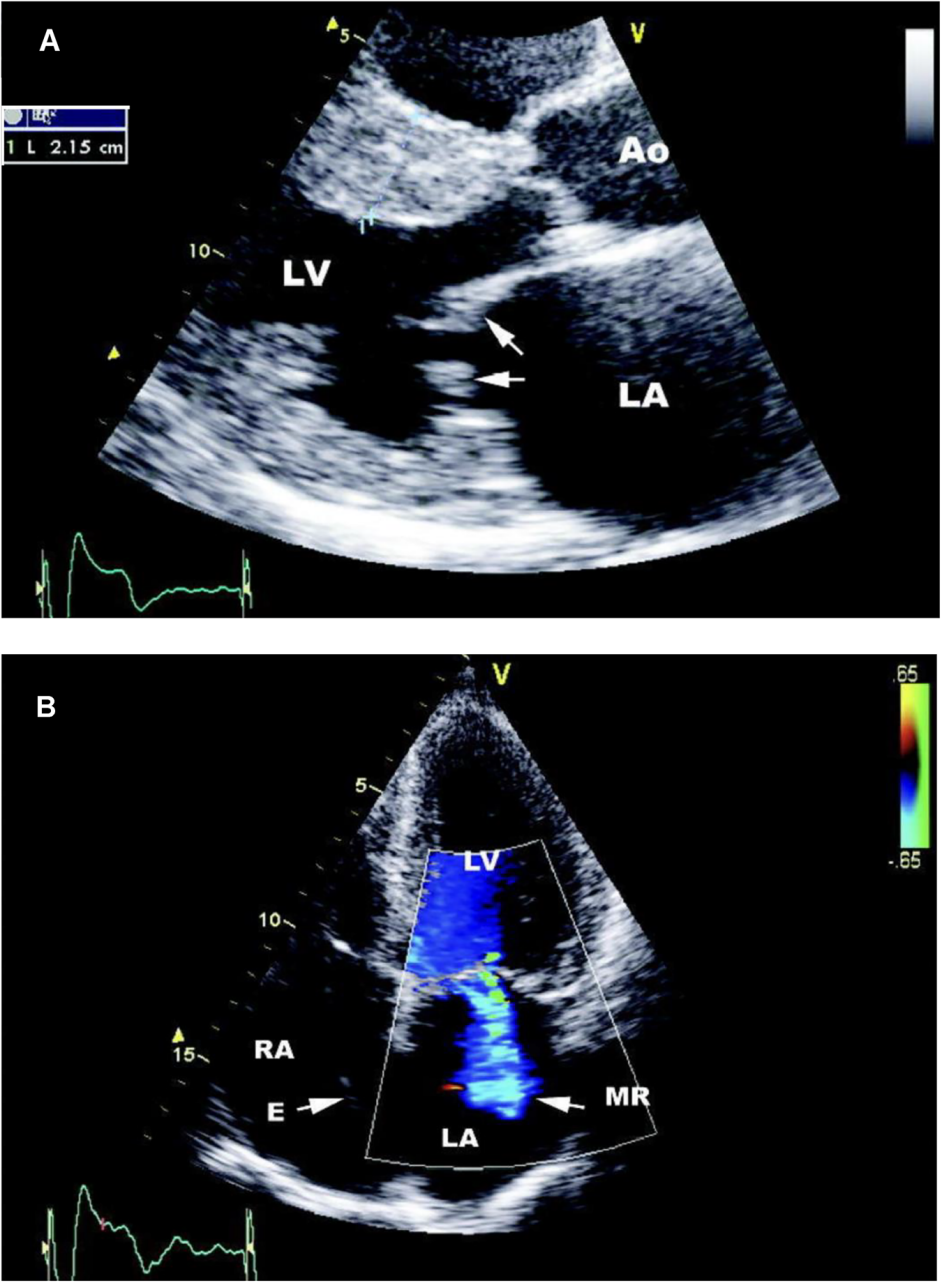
Echocardiographic findings in Fabry disease. A Echocardiogram showing marked interventricular sepal thickening (2.15 cm) and thickening of the MV (white arrows); B Echocardiogram with colour Doppler showing mild-moderate MR. Reproduced with permission from Linhart^81^. MV mitral valve, MR mitral regurgitation, LA left atrium, RA right atrium.
